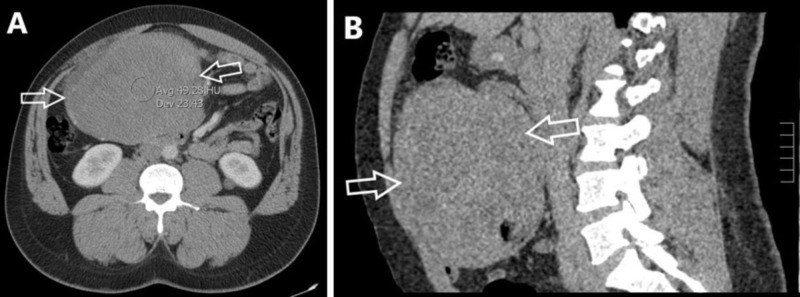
CT imaging with intravenous contrast of the abdomen and pelvis. Axial (A) and sagittal (B) CT imaging planes showing a large intra-abdominal mass (white arrows).
